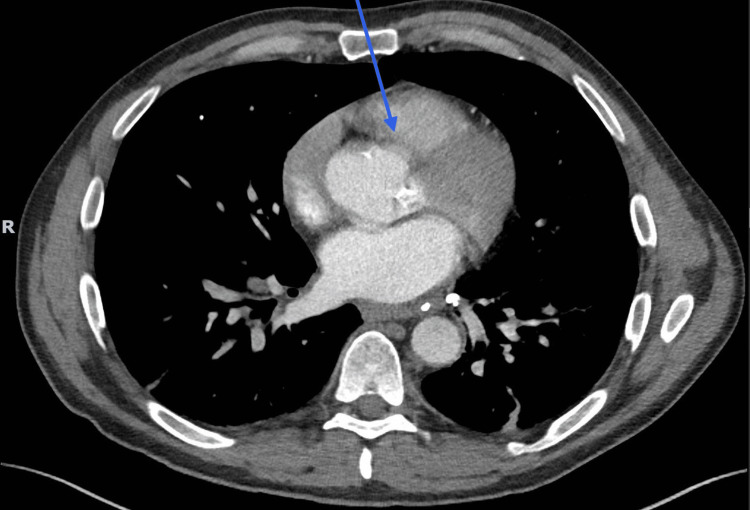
CTA A CTA demonstrated tricuspid vegetations and an irregular atherosclerotic plaque/atheroma in the ascending aorta. Superimposed vegetation involving the aortic plaque could not be excluded. No definite vegetation was visualized on the bicuspid aortic valve on the CTA. CTA, CT angiogram
